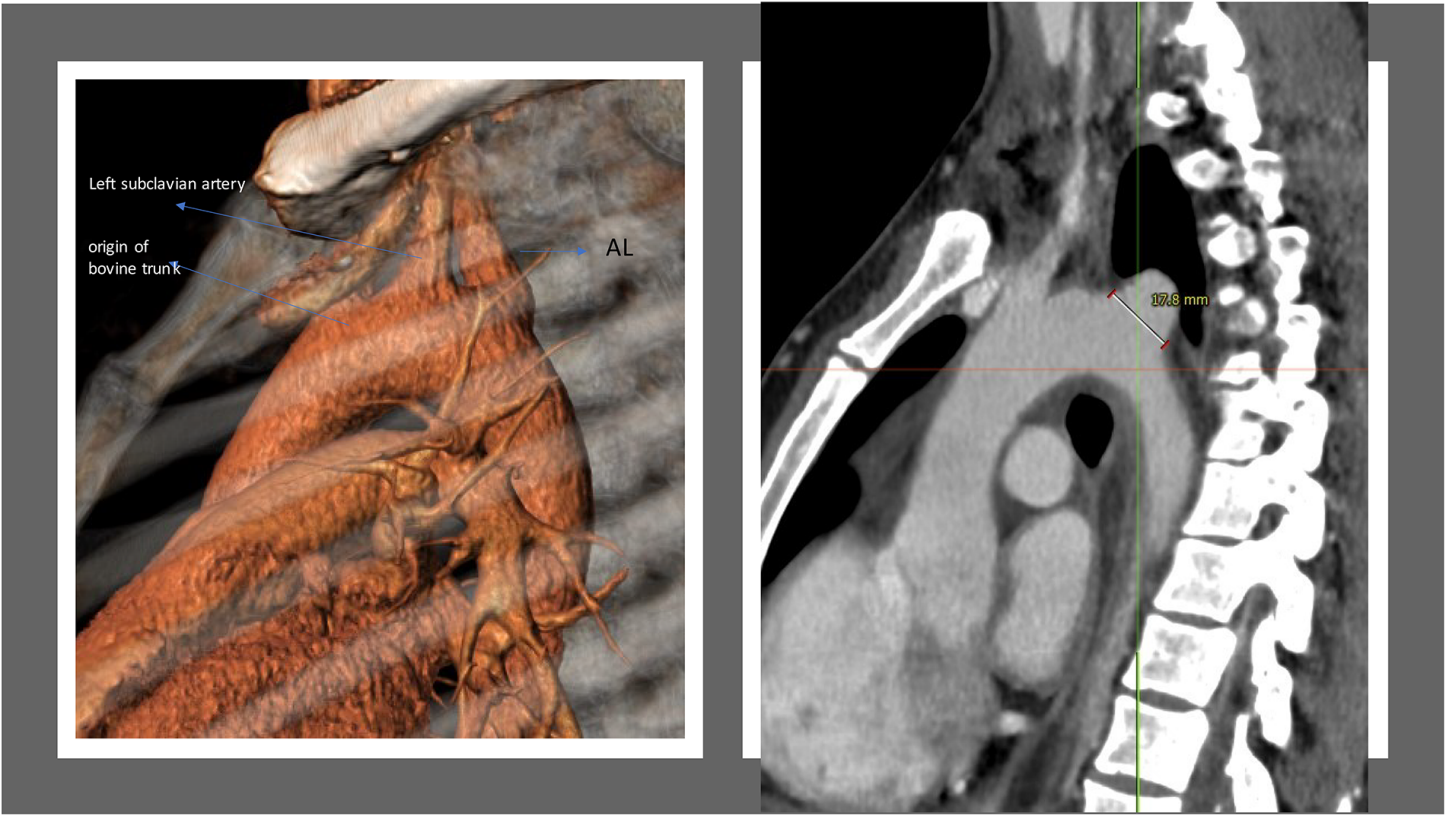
3-Dimension reconstruction and sagittal CT scan view revealing an aberrant origin of the right subclavian artery from the aortic arch and a dilated appearance of its proximal segment (17.8 mm), with a retro-esophageal course.
